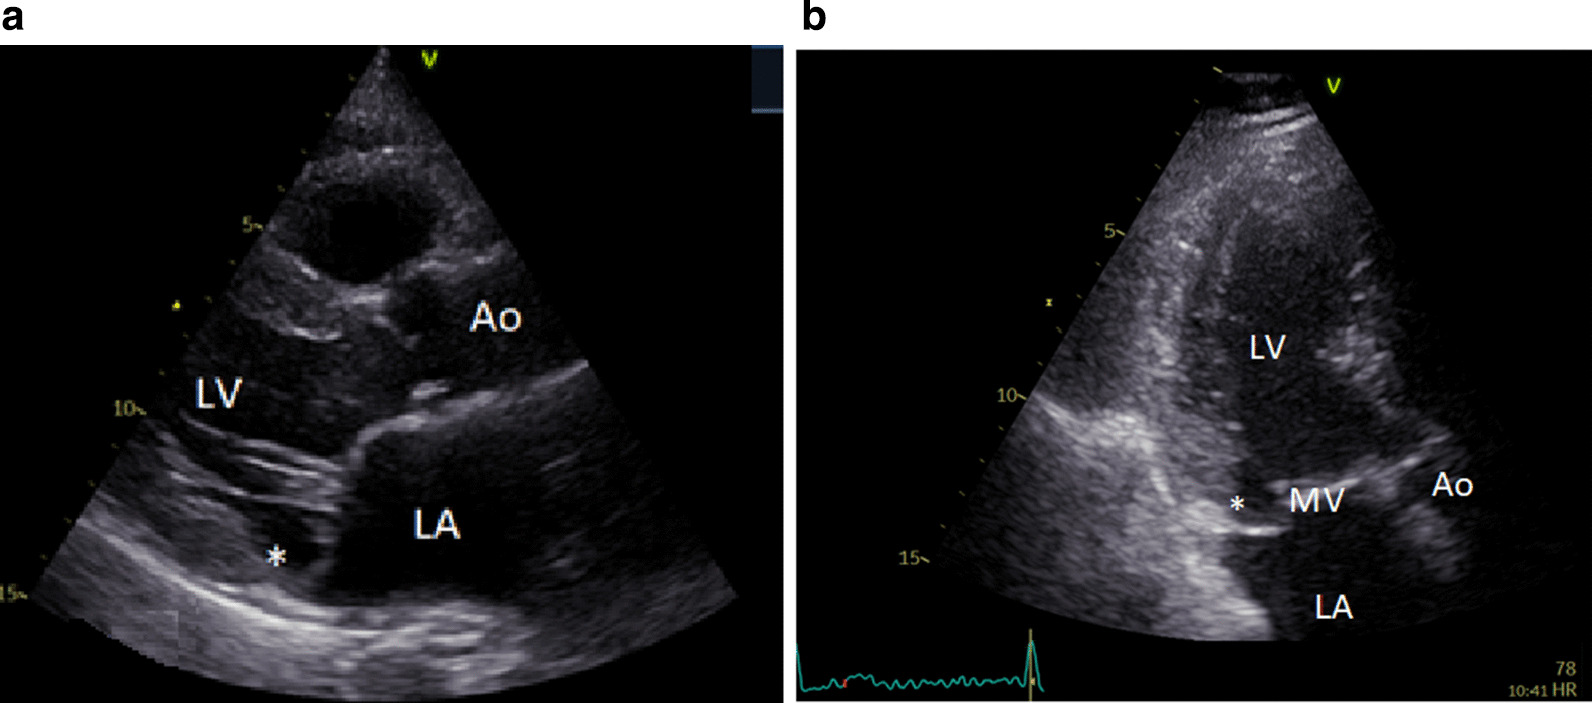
Transthoracic echocardiographic imaging of mitral annular disjunction. Mitral annular disjunction (*) as seen during ventricular on transthoracic echocardiography in the parasternal long axis view (a) and the apical three chamber view (b). *Ao* aorta, *LA* left atrium, *LV* left ventricle, *MV* mitral valve, *RV* right ventricle
